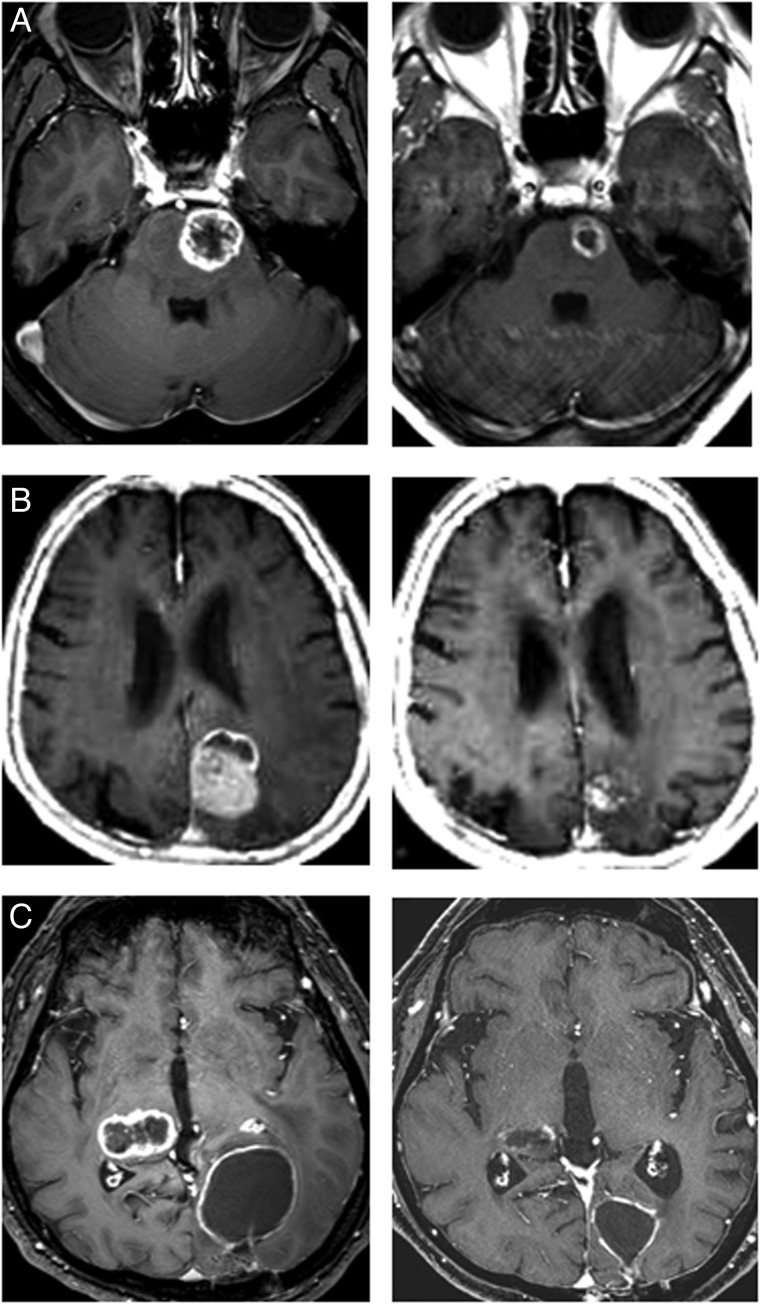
Gd-enhanced T1-weighted MR images. (A) Cecal cancer brain metastases in a 43-year-old male. A pons tumor was treated with a marginal dose of 31 Gy in five fractions at a 51% isodose (left). A significant tumor response and no adverse imaging effects were found five months after the five-fraction CyberKnife radiotherapy (right). The right hemiparesis disappeared and the KPS improved from 60 to 70. (B) A lung cancer (non-small-cell) brain metastasis in a 78-year-old male. A tumor in the occipital lobe with perifocal edema was treated with a marginal dose of 31 Gy in five fractions at a 54% isodose (left). A tumor response was found eight months after five-fraction radiotherapy (right). (C) Lung cancer (non-small-cell) brain metastases in a 53-year-old male. A tumor in the visual area with perifocal edema was treated with a marginal dose of 29 Gy in five fractions at a 53% isodose, and a right thalamic tumor was treated with a marginal dose of 27 Gy in three fractions at a 54% isodose (left). A tumor response was found seven months after treatment. Both tumors decreased in size and no adverse effects were observed (right).
